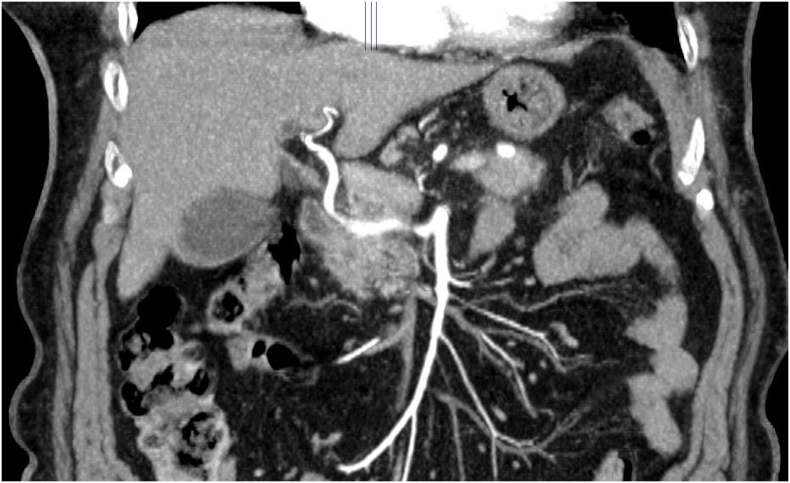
CT image shows the replaced CHA.
